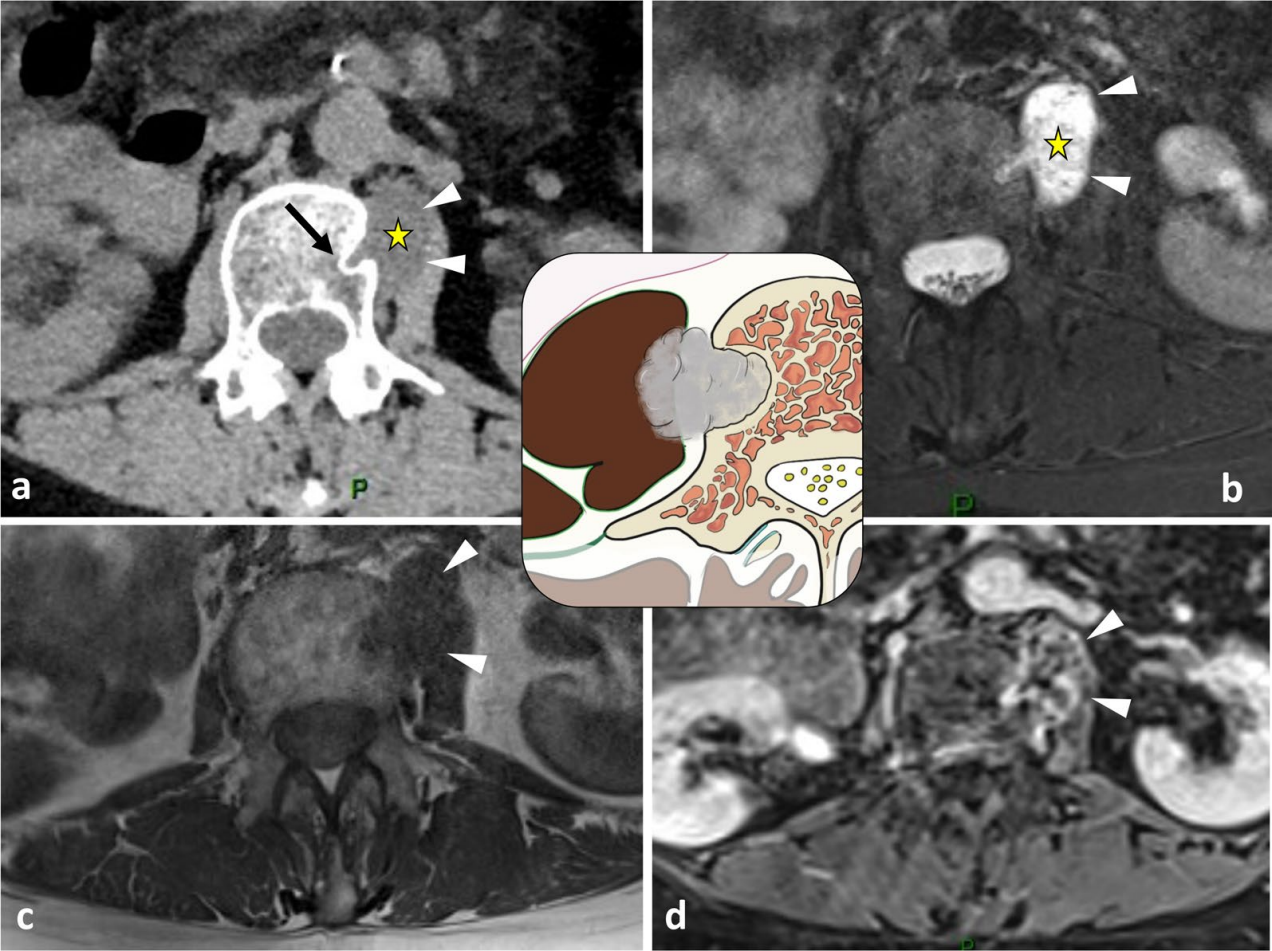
Schwannoma* of a ventral branch of the right 2nd lumbar spinal nerve in a 32-year-old female with neuropathic pain of the right anterior medial thigh. Axial CT reconstruction (a) shows a hypodense mass within the psoas muscle (white arrowhead) with high signal on T2WI (b), low signal on T1WI (c) and heterogeneous contrast-enhanced fat saturation T1WI (d). The mass extends into the vertebral body forming a lytic lesion with sclerotic margin (black arrow). The yellow star shows the epicentre of the mass
